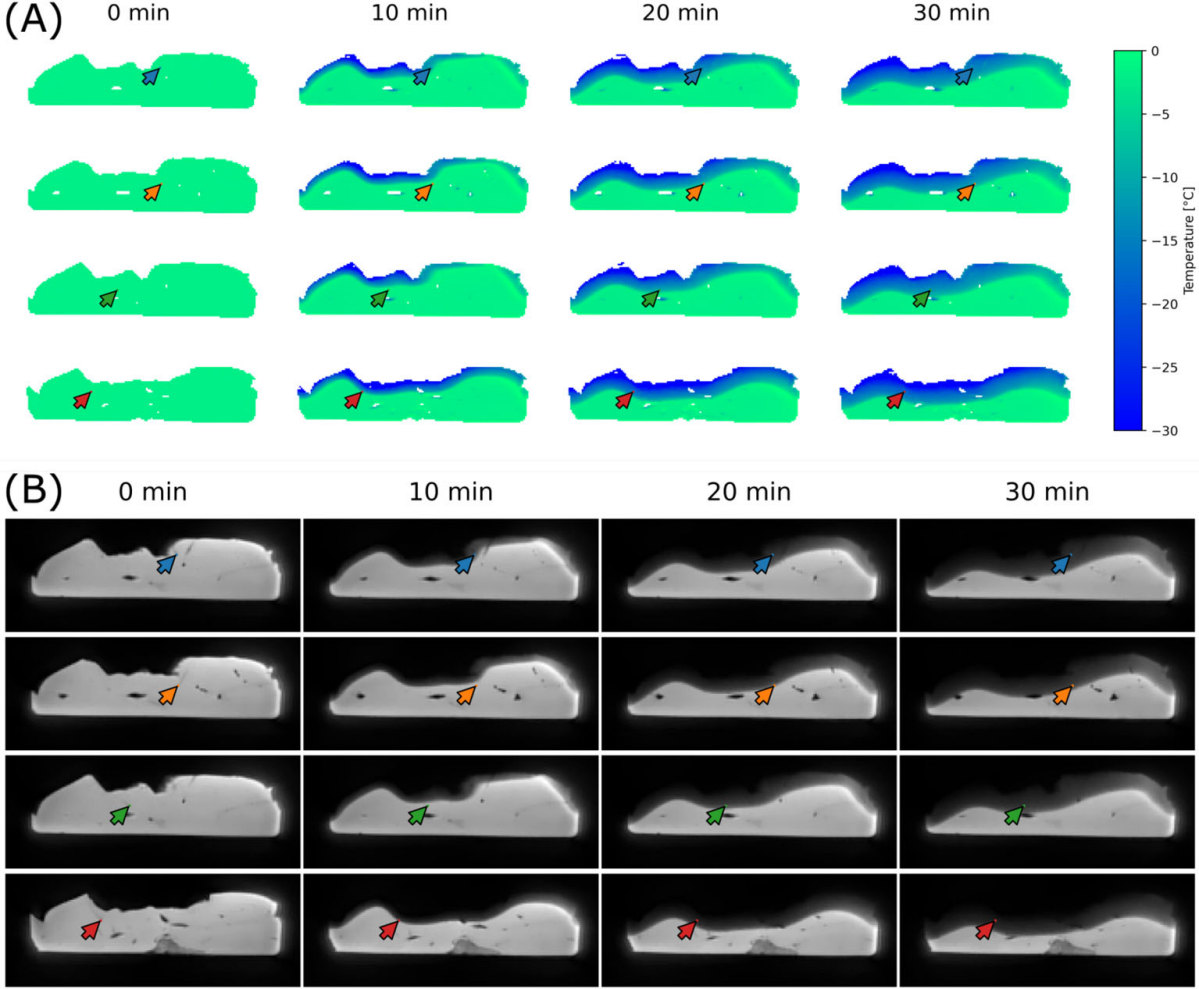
Axial slices at the location of the temperature sensors. (A) MRI‐estimated temperature maps and (B) corresponding magnitude images acquired at 0, 10, 20, and 30 min into the experiment. Sensor positions are indicated. Note that temperatures above 0°C cannot be estimated by the used method and appear to be close to 0°C.
